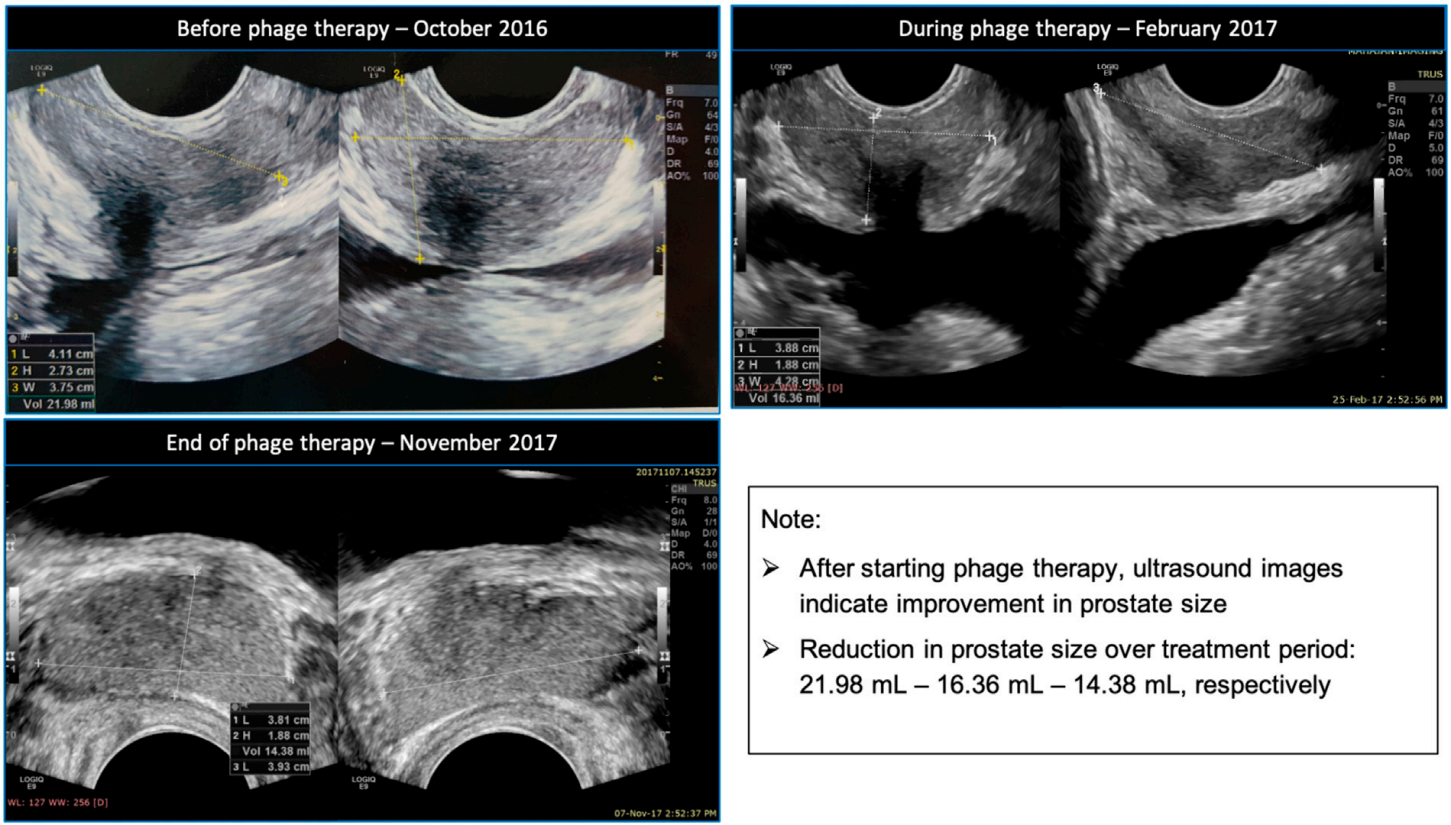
Clockwise ultrasound images of the patient’s prostate before, during and towards the end of his phage therapy.
